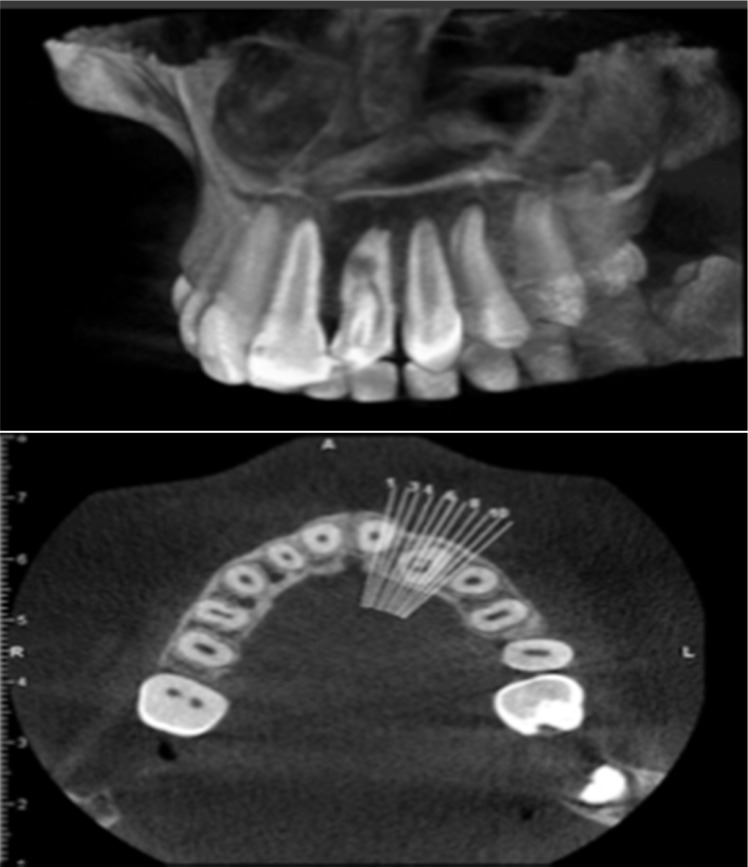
CBCT was used for optimal diagnosis that revealed two separate canals. The invaginated canal was almost at the center of the root but the other canal was located mesiolingually
